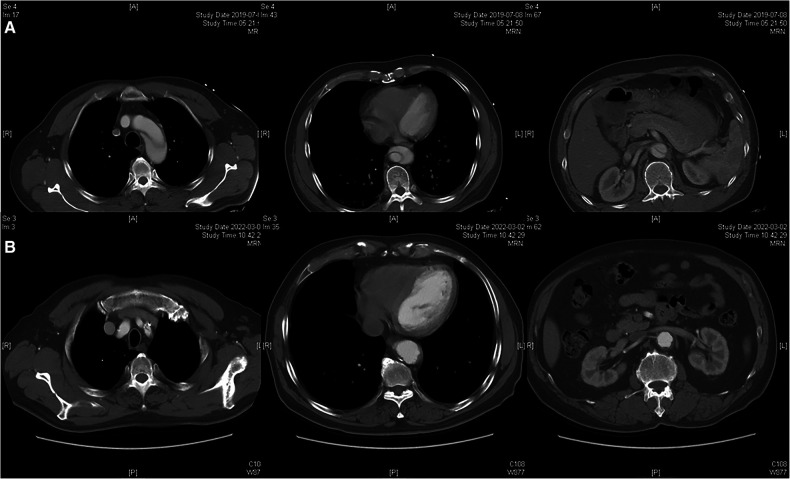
Radiographic image of fenestration technique. (A) Preoperative CTA showed the dissection involving the distal aortic arch and visceral aortic segment. (B) Postoperative CTA indicated the patency of the LSA and complete thrombosis of the visceral aortic segment over 2 years.
